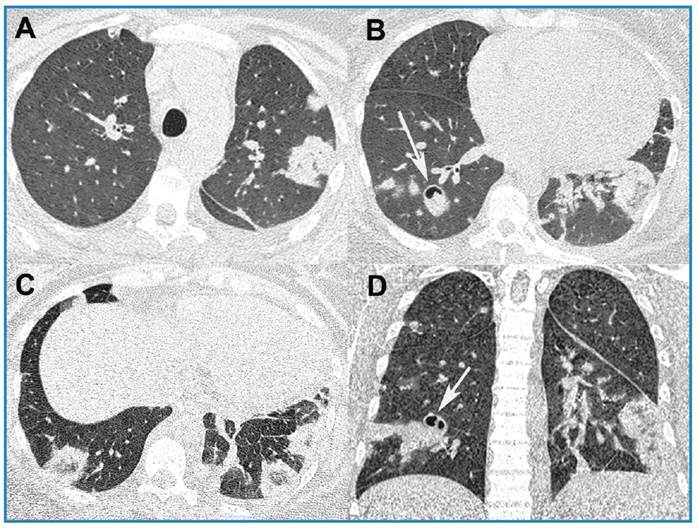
Chest computed tomography images obtained five days after the first examination in the same planes. demonstrating partial resolution of lesions, some of which show cavitation (arrows).
